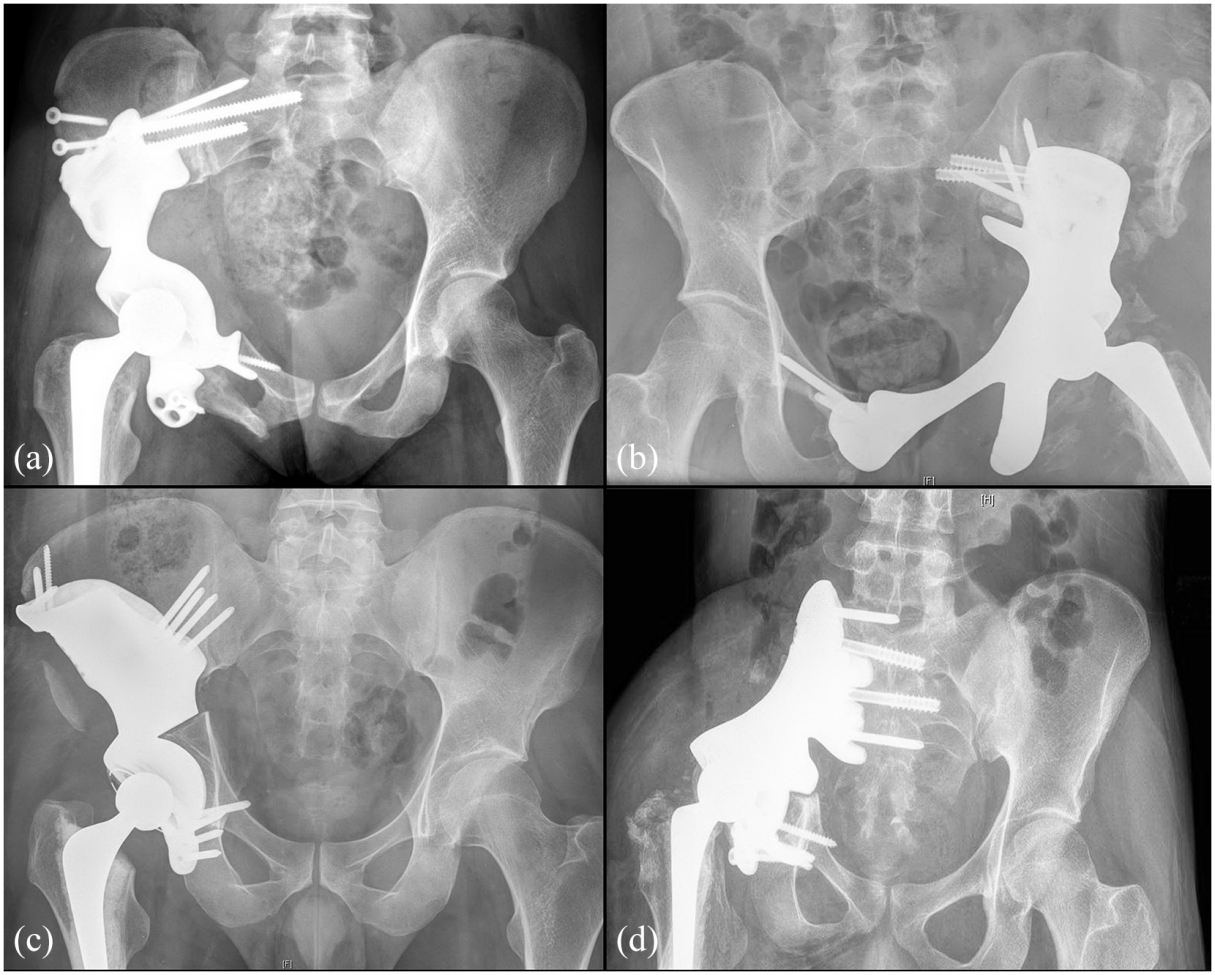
Four post-operative antero-posterior x-rays of a pelvis showing the implantation of a 3DPPI for (a) P1-2-3 resection for metastatic breast carcinoma, (b) P2-3 resection for chondrosarcoma, (c) P1-2 resection for metastatic testicular carcinoma, and (d) P1-2 resection for recurrent Ewing sarcoma.
